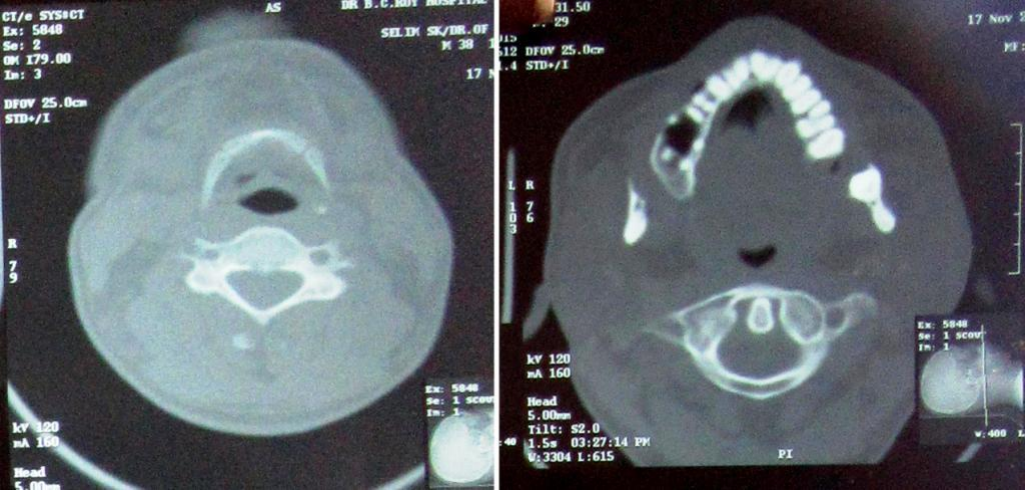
Axial section of computed tomographic image showing almost completely resorbed lower arch along with hypodense areas in right upper maxilla.
